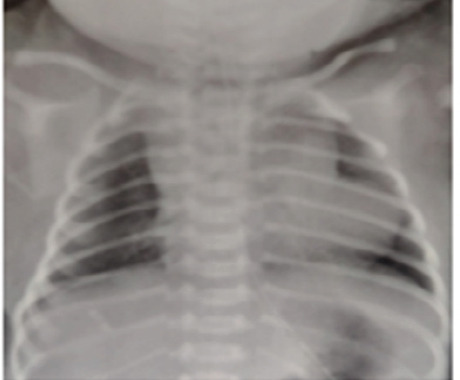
Chest X-ray showing boot shaped heart.
